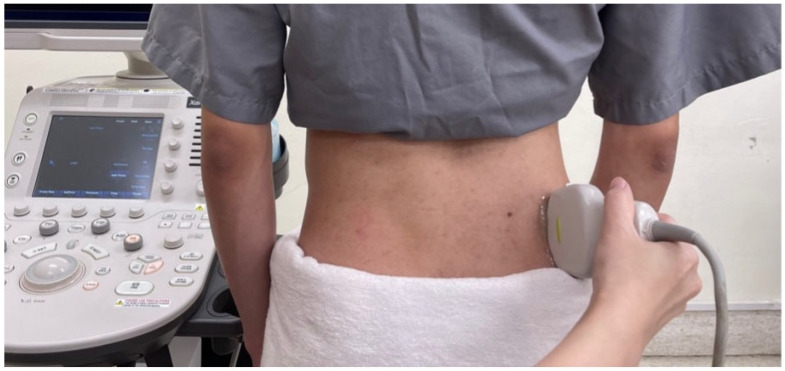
Location of GM ultrasonographic.
